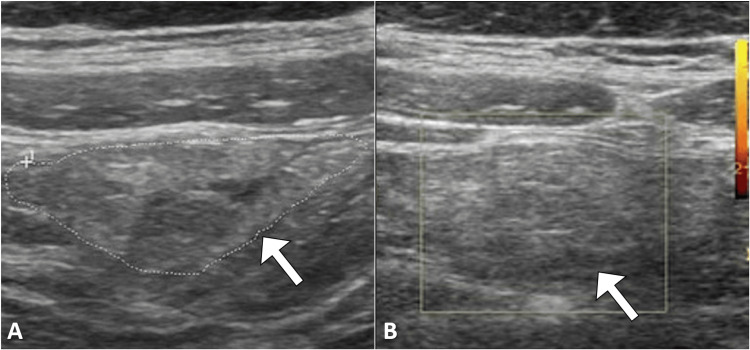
Gray-scale ultrasound image (A) shows a focal area of omental fat with increased echogenicity (arrow), while the corresponding color Doppler image (B) reveals no blood flow.
